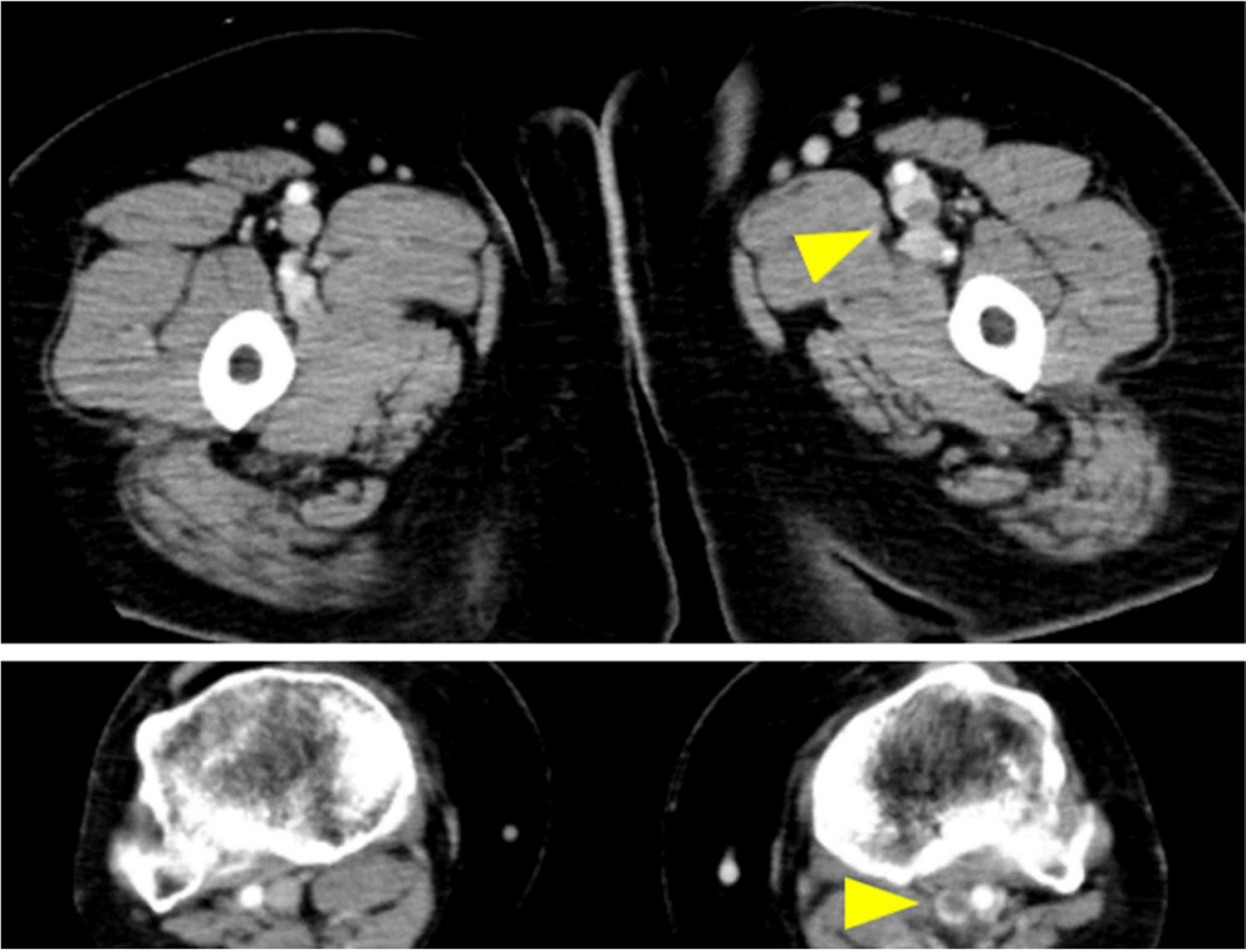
Contrast-enhanced computed tomography reveals occlusive intravenous thrombosis in the left popliteal vein and the left superficial femoral vein (yellow arrowheads)
